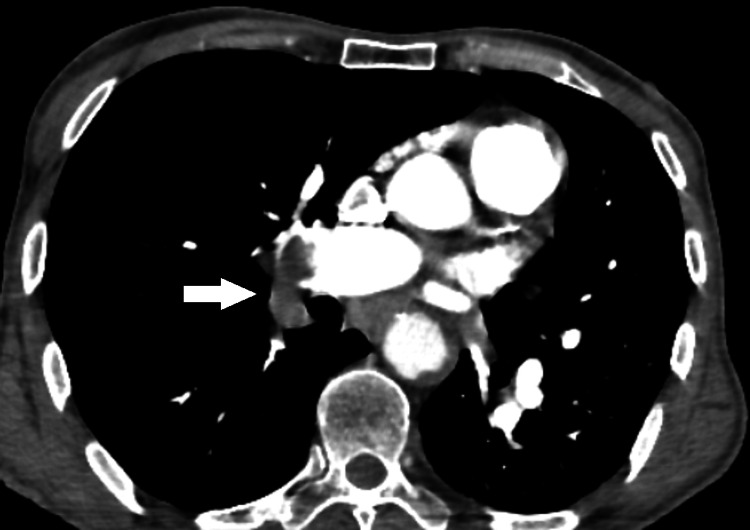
CT pulmonary angiography This axial section, in arterial time, shows hyperdense material within the right pulmonary artery extending to its lobar branches corresponding to the thrombus.
